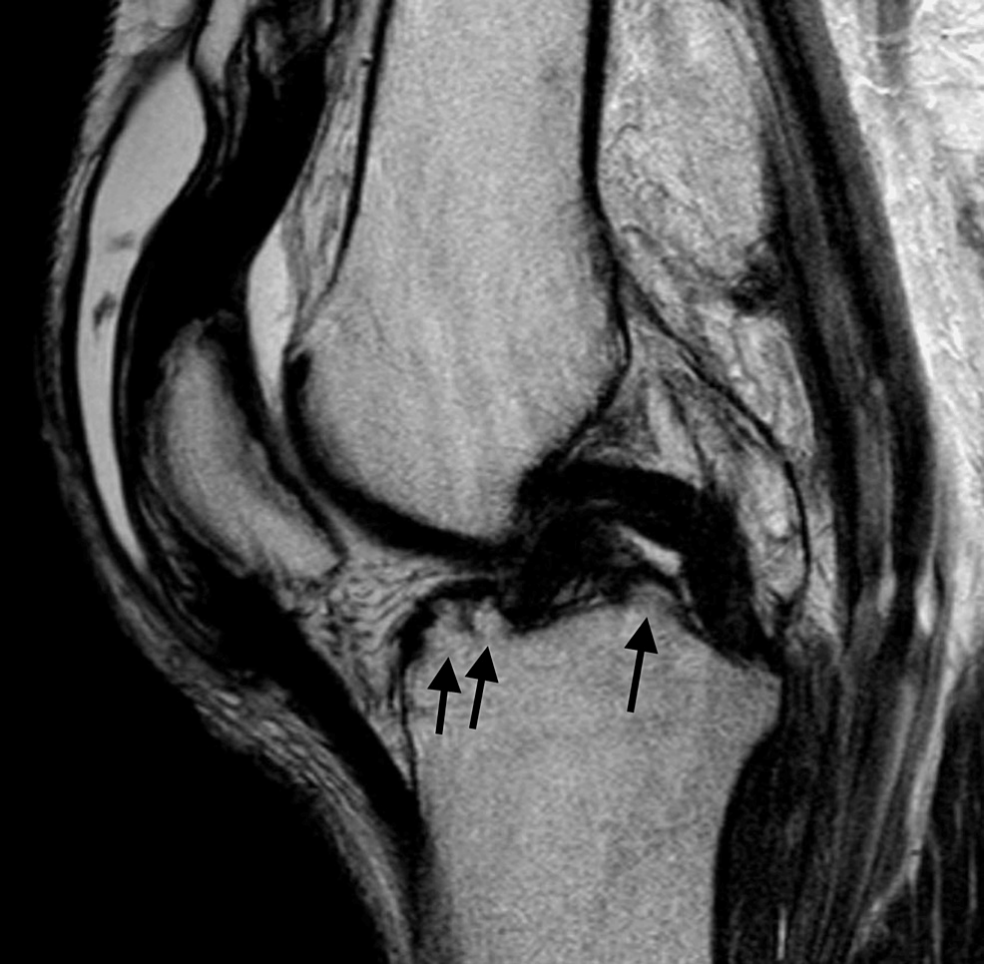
The MRI of the bilateral knees shows pre-patellar soft tissue swelling and possible intra-articular loose bodies (arrows). MRI: magnetic resonance imaging
